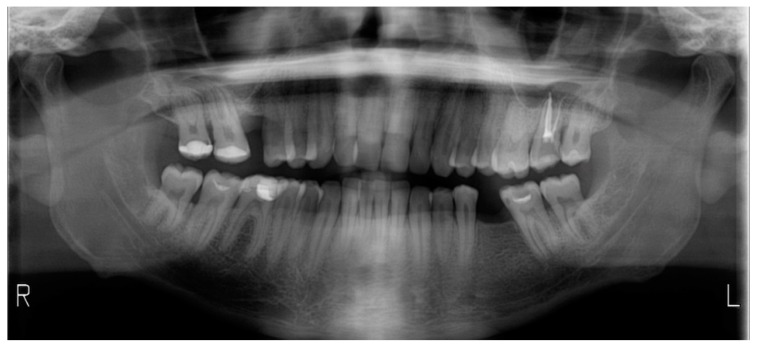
Case 2: orthopantomography.
